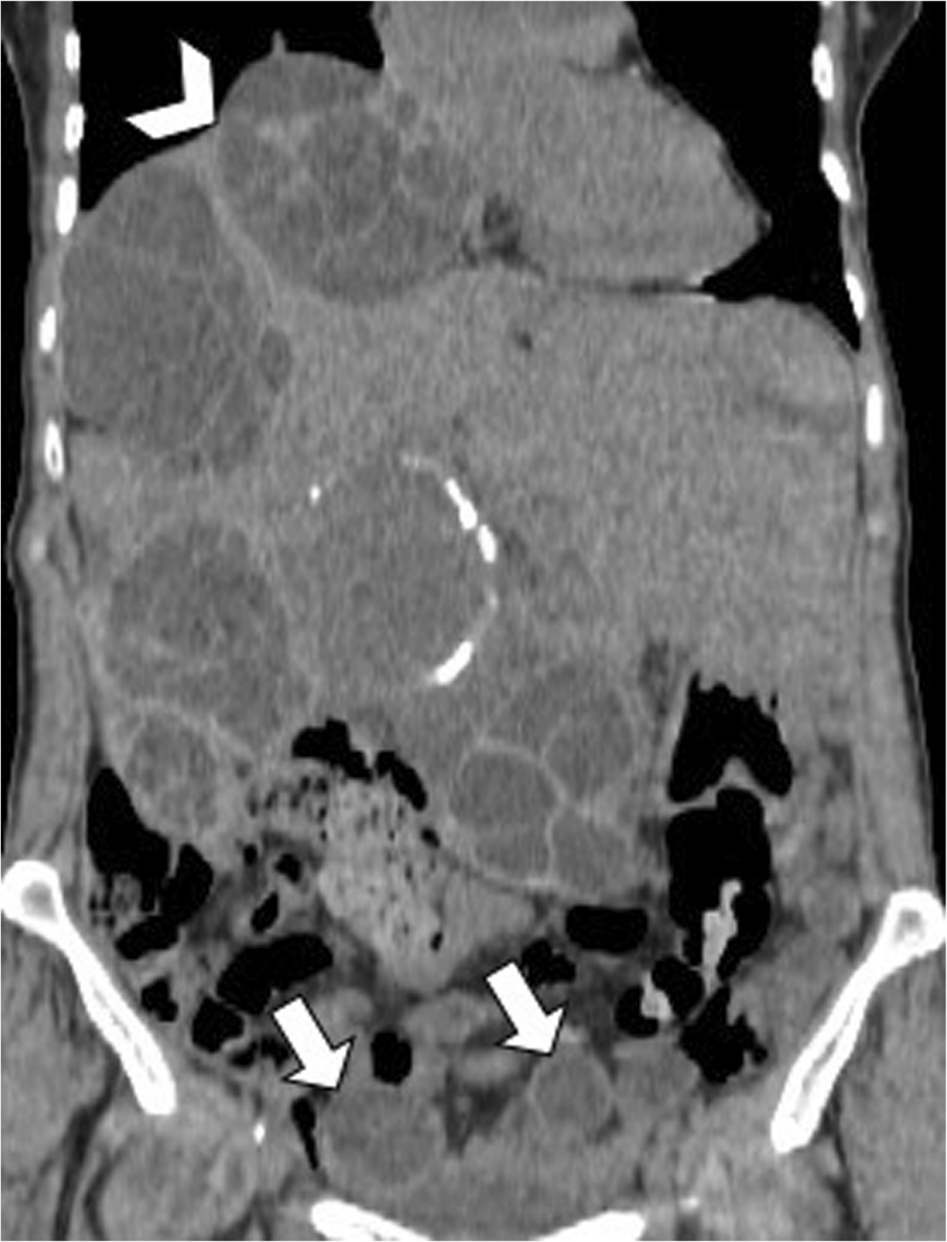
A 66-year-old woman with diffuse abdominal echinococcosis. Coronal reformatted CT image shows peritoneal dissemination of hydatid cysts (arrows), due to direct rupture of the original liver cysts and compression of the right diaphragm by one of the hepatic hydatid cyst (arrowhead)
